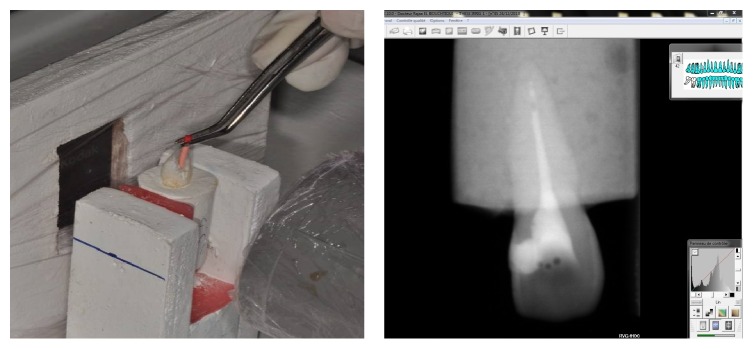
Active irrigation stirring of the cone and the corresponding radiograph.
